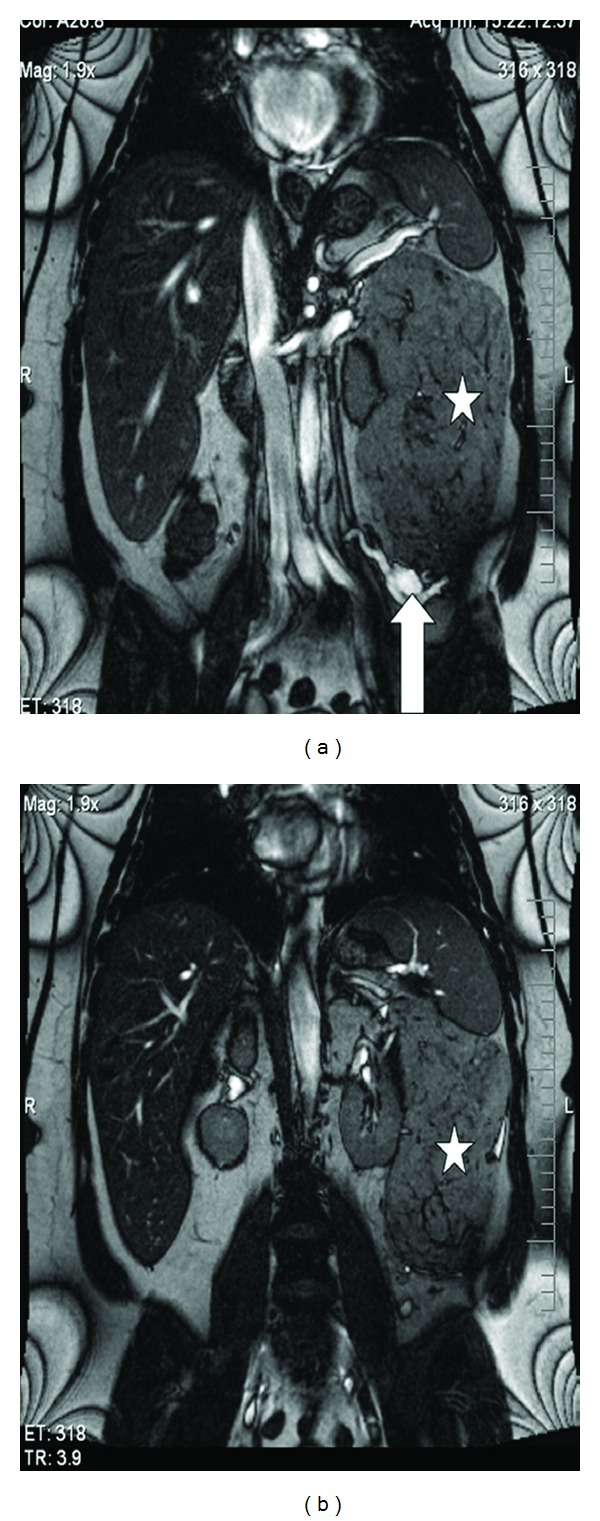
Abdominal magnetic resonance imaging demonstrating a large fatty encapsulated mass (white asterisk) measuring 19.3 cm × 13.5 cm × 10.7 cm with prominent vascularity (white arrows). The anatomic relationship between the mass and the left kidney can be well seen in Figure 2(b).
